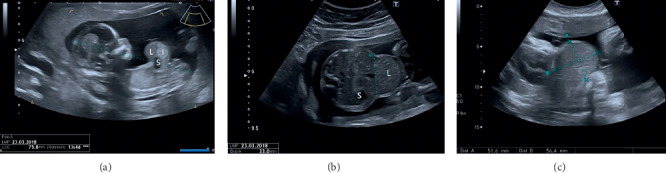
Ultrasound images of the omphalocele. (a) 13 + 4 weeks ultrasound: in the first trimester, the omphalocele contained the liver (L), the intestine (I), and part of the stomach (S). (b) 33 weeks ultrasound: the content of the omphalocele became only hepatic (L), being the stomach (S) correctly situated at the left side of the abdomen. (c) 37 weeks ultrasound: estimation of the final size of the omphalocele before the elective caesarean section.
